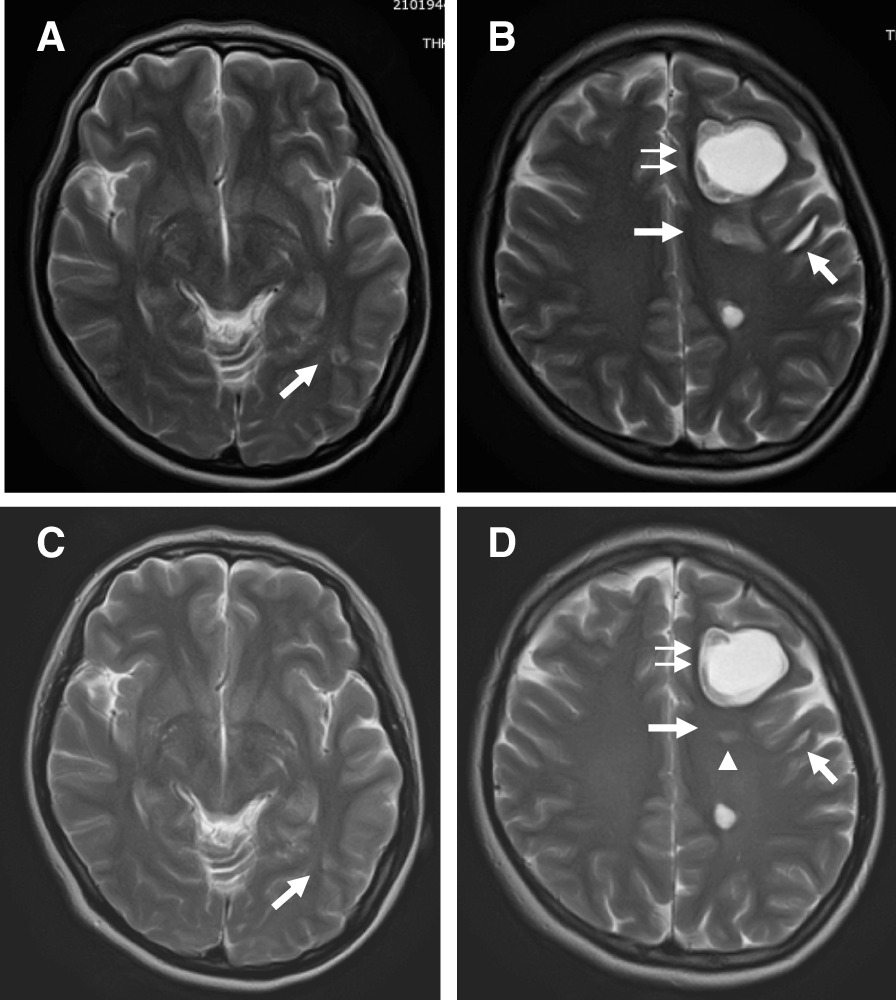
T2-weighted brain MRI scans obtained 7- (A, select all B) and 30-days (C, D) after the start of cysticidal treatment. Except for the largest, the size of almost all lesions in the frontal lobe was decreased as was perilesional edema (arrows). There is no change in the left frontal lesion (double arrows). The scolex inside the cyst located outside the left lateral ventricle has disappeared (arrowhead)
